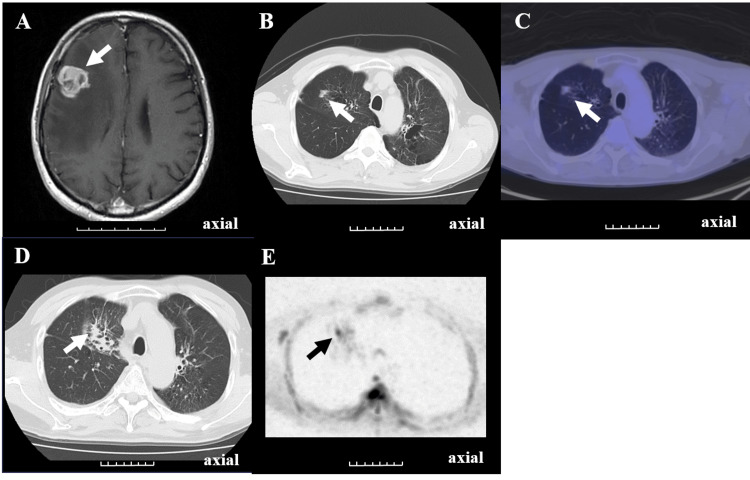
Brain MRI, chest CT, PET-CT, DWIBS images (A) Contrast-enhanced T1-weighted magnetic resonance imaging (MRI) reveals a 2.5cm ring-shaped contrast-enhanced lesion in the right frontal lobe, suggesting brain metastasis (white arrow); (B) Initial chest CT reveals emphysematous changes in the right lung apex (white arrow); (C) while fludeoxyglucose-18 positron emission tomography (FDG-PET) shows no uptake of fludeoxyglucose-18 (SUVmax: 1.3) in the same area (white arrow); (D) Chest computed tomography (CT) performed four months after initial chest CT demonstrates enlargement of the lesion in the right lung apex, suggesting lung cancer (primary tumor) (white arrow); (E) Diffusion-weighted whole-body imaging with background body signal suppression (DWIBS) with b=800 s/mm² performed four months after the initial chest CT shows high signal intensity (black on this image) in the same area (black arrow). The lesion is indicated in each panel by an arrow. Each division on the scale bar is 1 cm.
